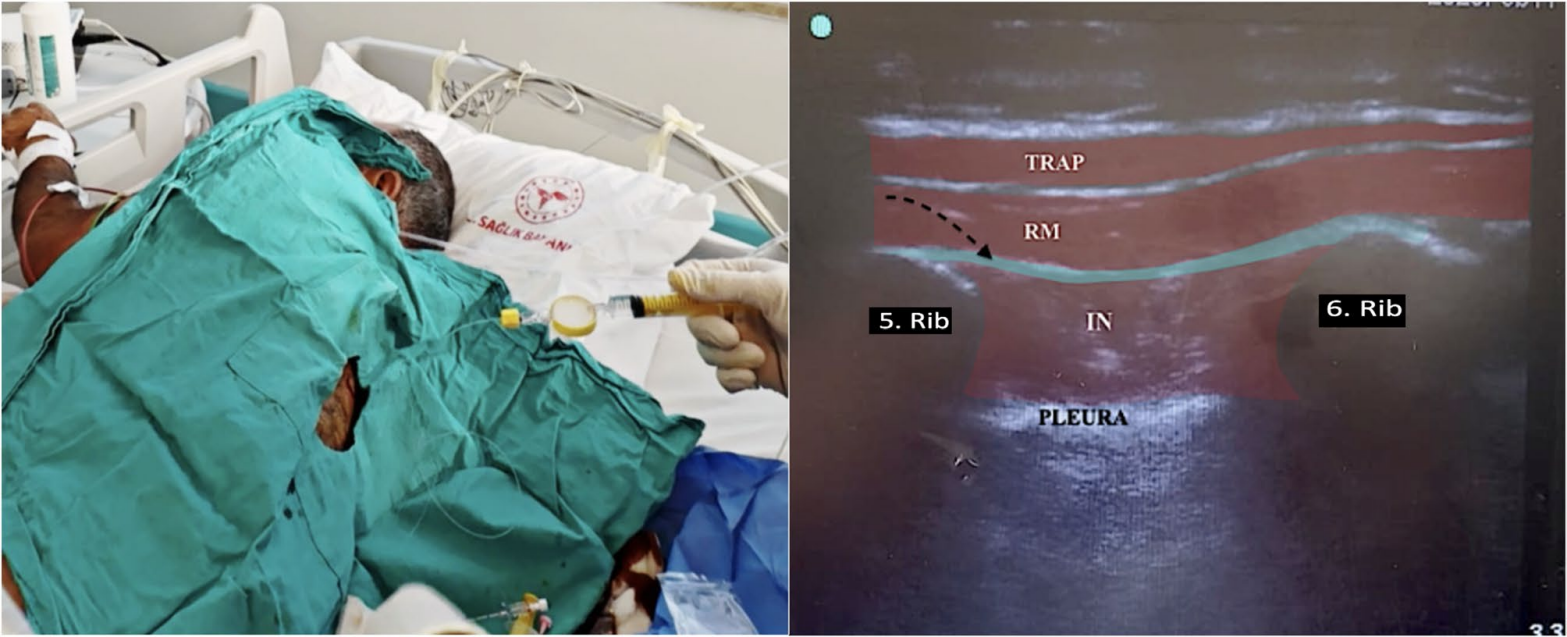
Ultrasound guided rhomboid intercostal plane block and placement catheterization. TRAP: Trapezius muscle RM: Rhomboid muscle IN: Intercostal muscle
